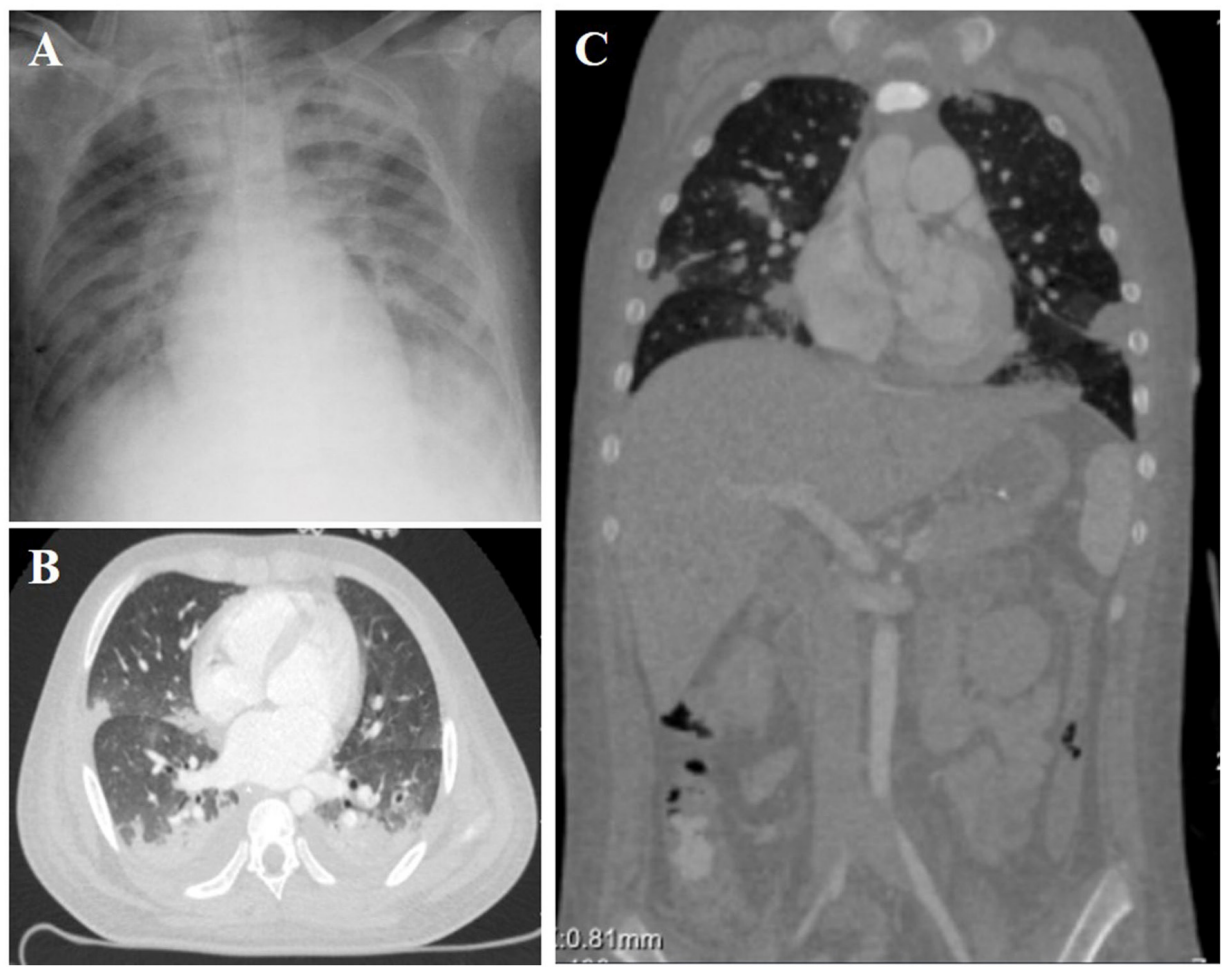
Chest X-ray (A) and Contrast-enhanced computed tomography (B,C) showed mild cardiomegaly, interstitial pulmonary edema, and bilateral pulmonary contusions.
